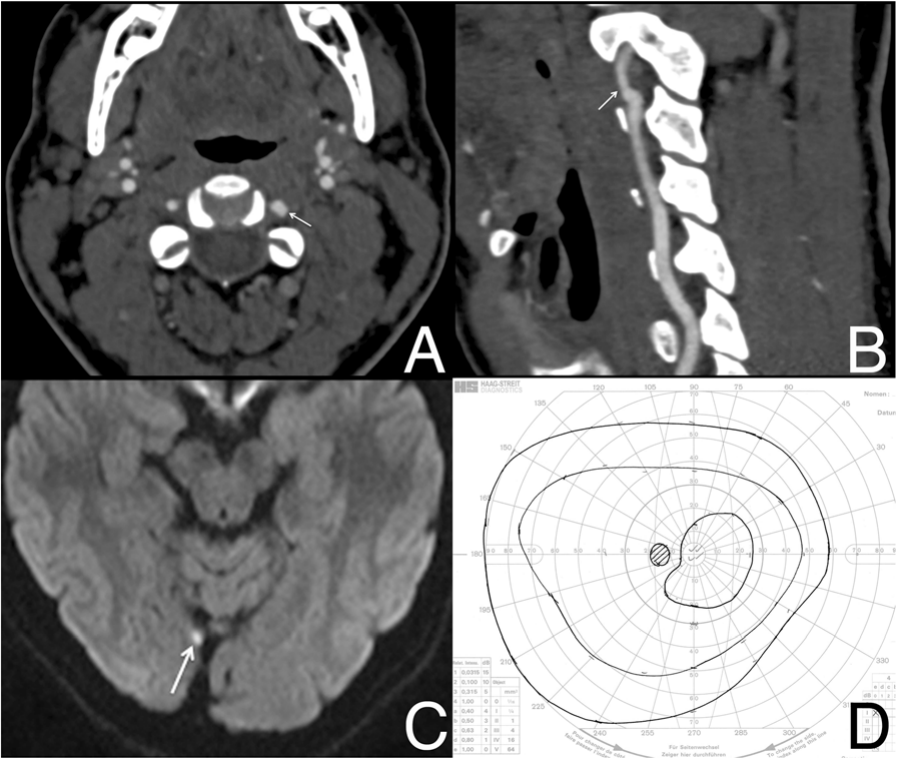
Diagnostic imaging and perimetry. a Computed tomography of head and neck (axial view) showing a mural irregularity in the V2 segment of the left (arrow) vertebral artery compared to the right. b Sagittal reformat showing the change in caliber of the lumen of the left vertebral artery (arrow). c Diffusion weighted (B1000) magnetic resonance image demonstrating the focal cortical infarct in the right occipital lobe (arrow). d Goldmann visual field showing no evidence of homonymous field defect
